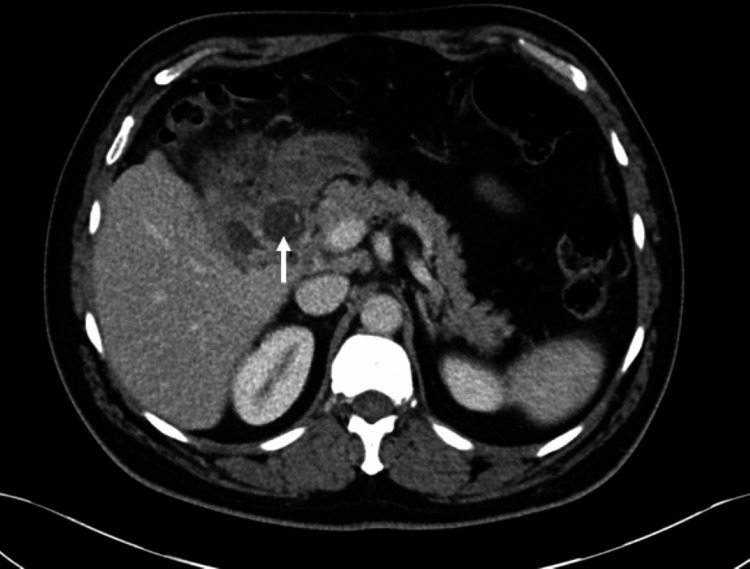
Axial portal venous CT shows a faintly calcified gallstone within the fistula (white arrow).
